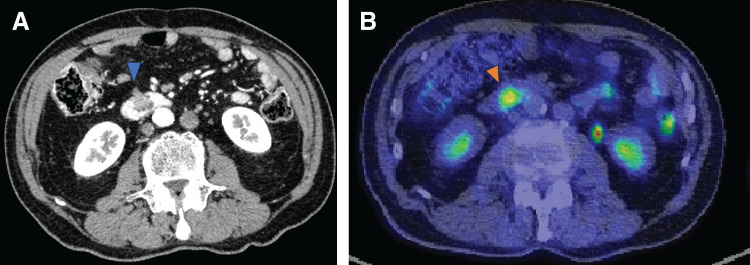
Preoperative findings of imaging examinations. A soft tissue shadow (blue triangle) was found at the duodenum on CT (A) and it (red triangle) showed strong accumulation of fluorodeoxyglucose on PET–CT scan (B).
